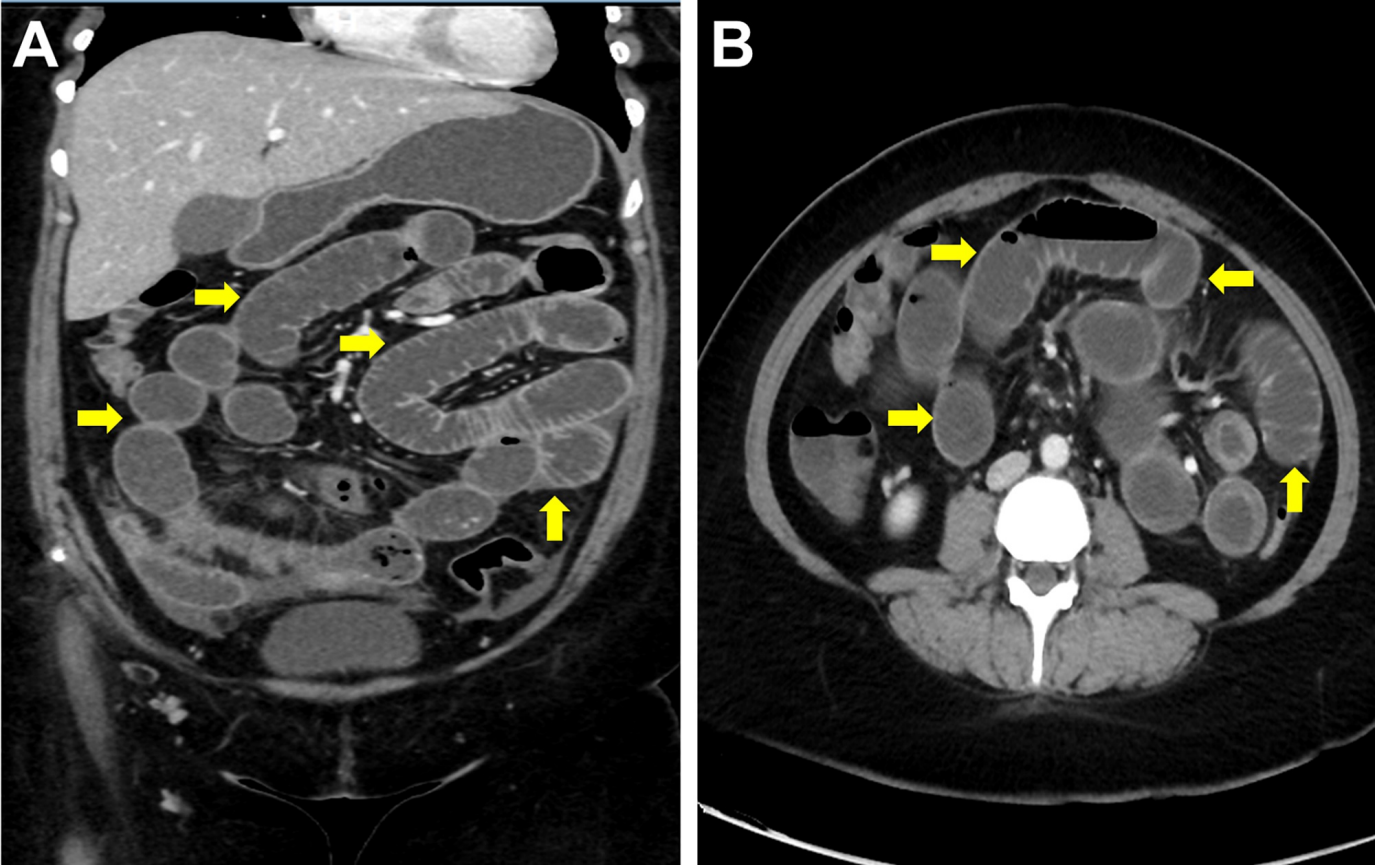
Coronal (A) and cross-sectional (B) contrast-enhanced computed tomography scans showing distended small bowel with a thickened edematous intestinal wall (arrows).
